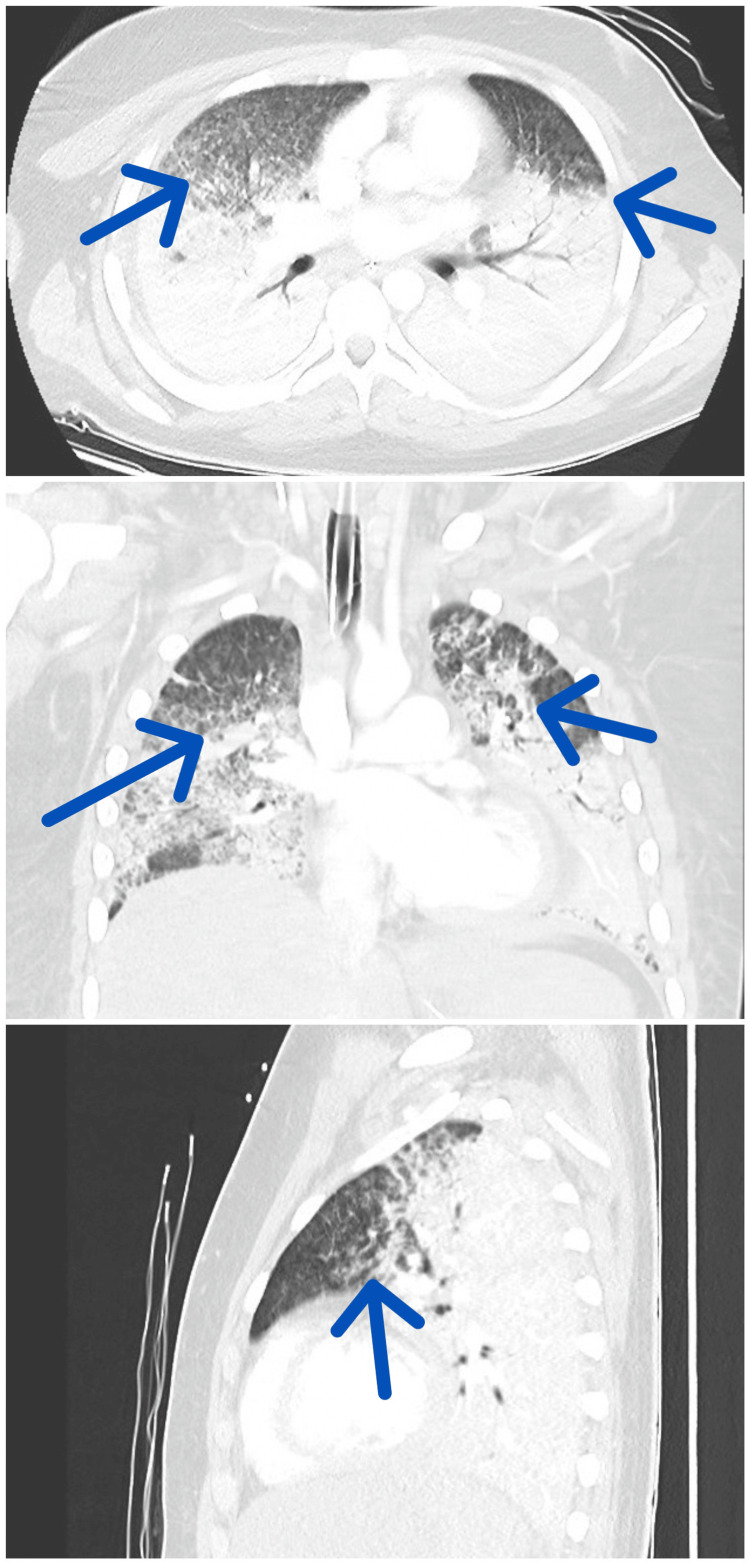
CT scan images suggestive of DAH (from top to bottom: axial, coronal, and sagittal views of the lung field) DAH, diffuse alveolar hemorrhage
